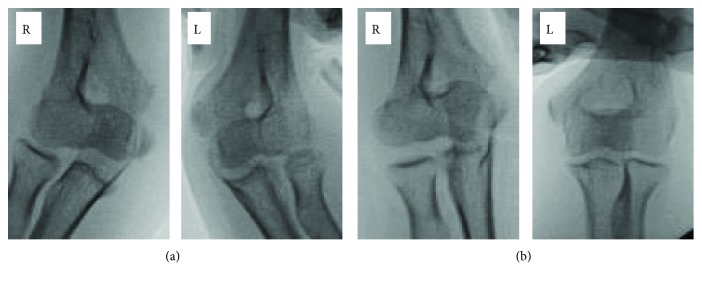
Preoperative stress X-ray of the bilateral elbow evaluated under anesthesia. (a) Valgus. (b) Varus. (R: right elbow, L: left elbow.) The side-to-side differences of the joint space widening were significantly worse in the right elbow.
